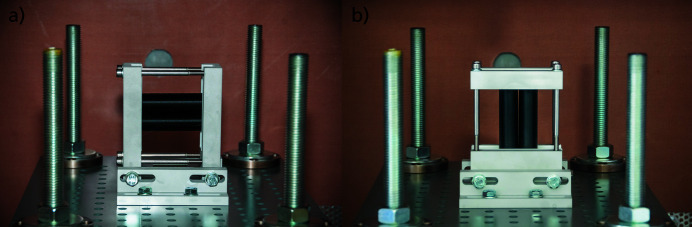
Image of the sample table including the sample holder. The dummy cells are shown in (*a*) a horizontal configuration and (*b*) a vertical configuration. The current setup includes variable positioning of the cell holder, height adjustment of the sample table and different configurable cell holders as well as two different positions for the nail devices: a flexible positioning of the initiation mechanism and high-speed X-ray radiography with cell configurations of up to four cylindrical cells.
